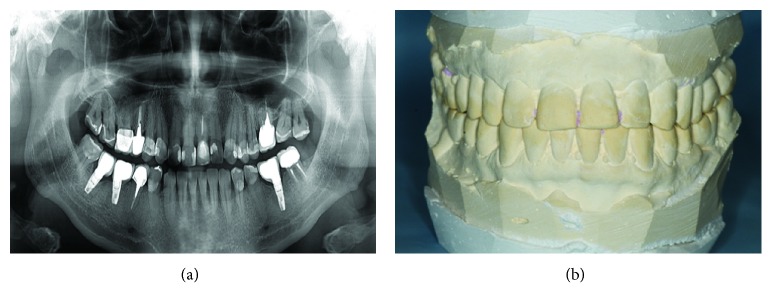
(a) Initial X-rays. (b) Initial study models.
